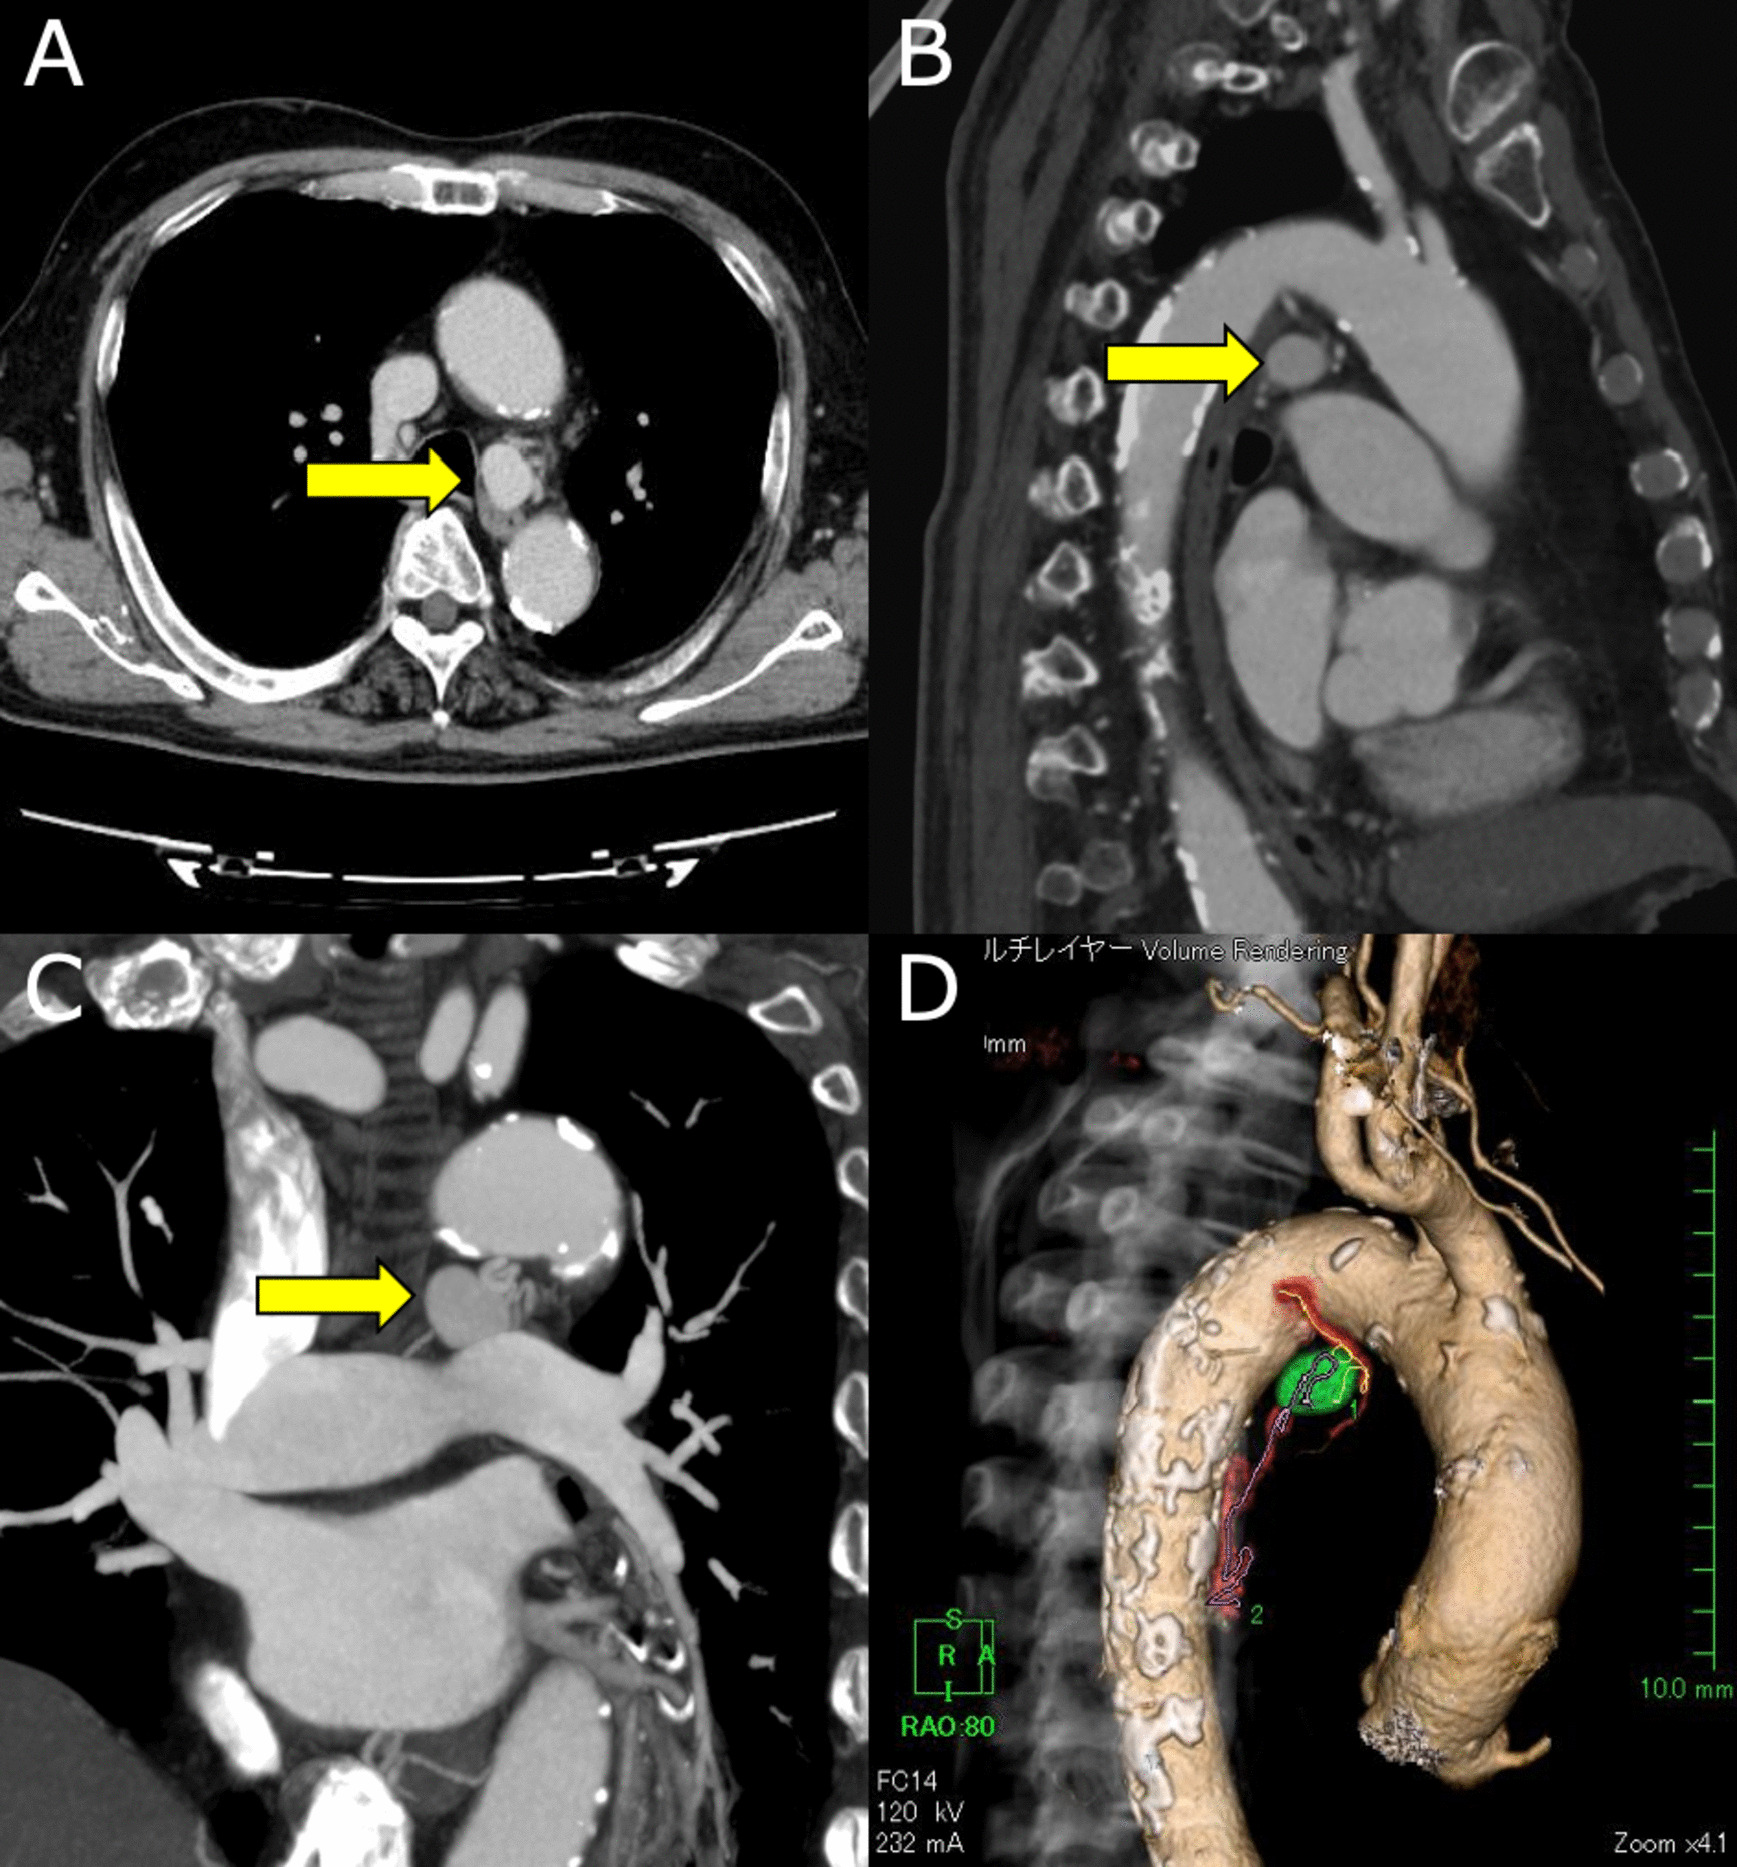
CECT images showing an aneurysm originating from the dorsal mediastinal side of the distal arch. A Axial view. B Sagittal view. C Coronal view. Yellow arrows in A, B, and C indicate the aneurysm. D Three-dimension CT shows an aneurysm (green) and an inflow artery (red)
